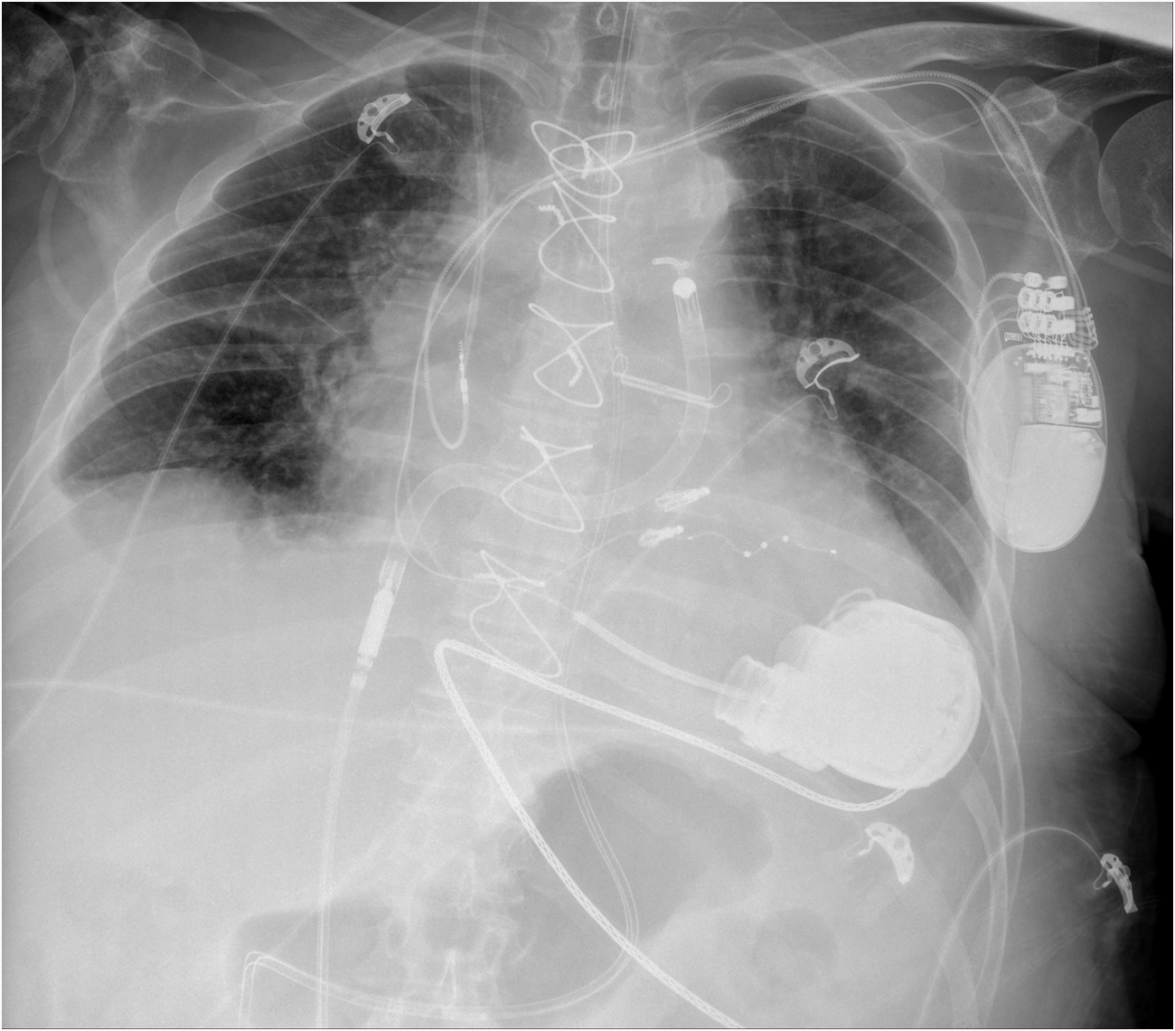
Bedside chest X-ray following implantation of the Impella RP system
